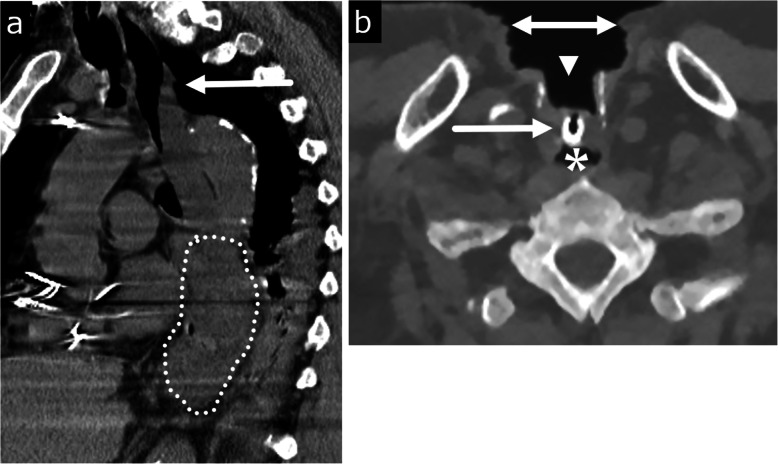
Esophageal obstruction with food bolus impaction (a) and therapeutic foreign body/prosthesis (b). a An 83-year-old demented male with chest pain during meal. Sagittal CT image shows a large chunk of food impaction in the mid to lower esophagus (dotted area). The upper esophagus is mildly dilated with an air-fluid level. b A 71-year-old male with history of laryngectomy for laryngeal cancer with a tracheoesophageal voice prosthesis which may mimic a foreign body. Axial CT image shows a small tubular shaped radiopaque device (arrow) between the tracheostomy (arrowhead) and the upper esophagus (asterisk). There is a tracheostomy defect and associated large skin defect anteriorly (bidirectional arrow)
